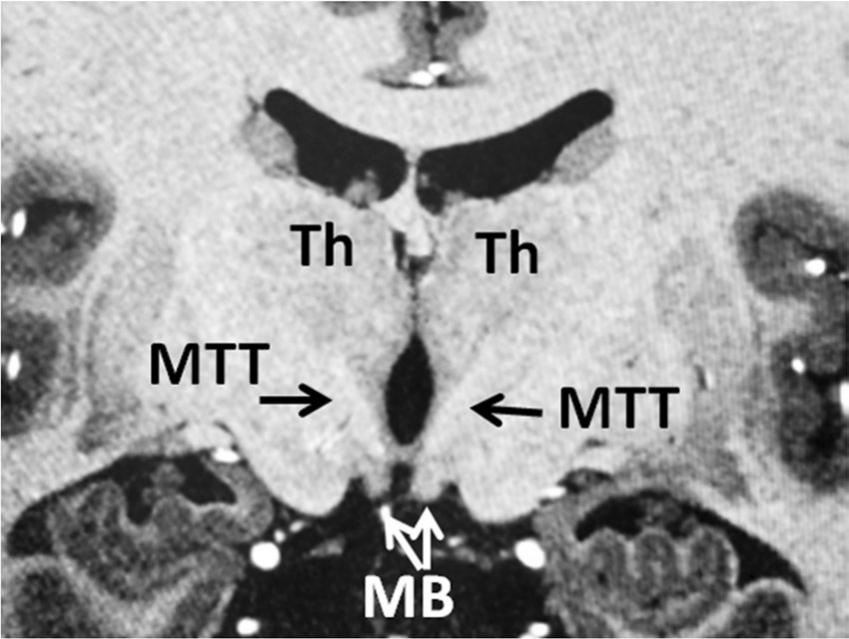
Coronal T1 weighted MRI showing the trajectory of bilateral MTTs on the coronal level just posterior to the mammillary bodies (MB). The bilateral MTTs are visible by T1 hypointense tracts coursing cranially toward the thalamus (Th) after exiting from the mammillary bodies. Please attention that the MTT already projected posteriorly before reaching this coronal level where it ascends into the thalamus. Lateral bendings of the MTTs are visible on this plane as they ascend within the thalami.
